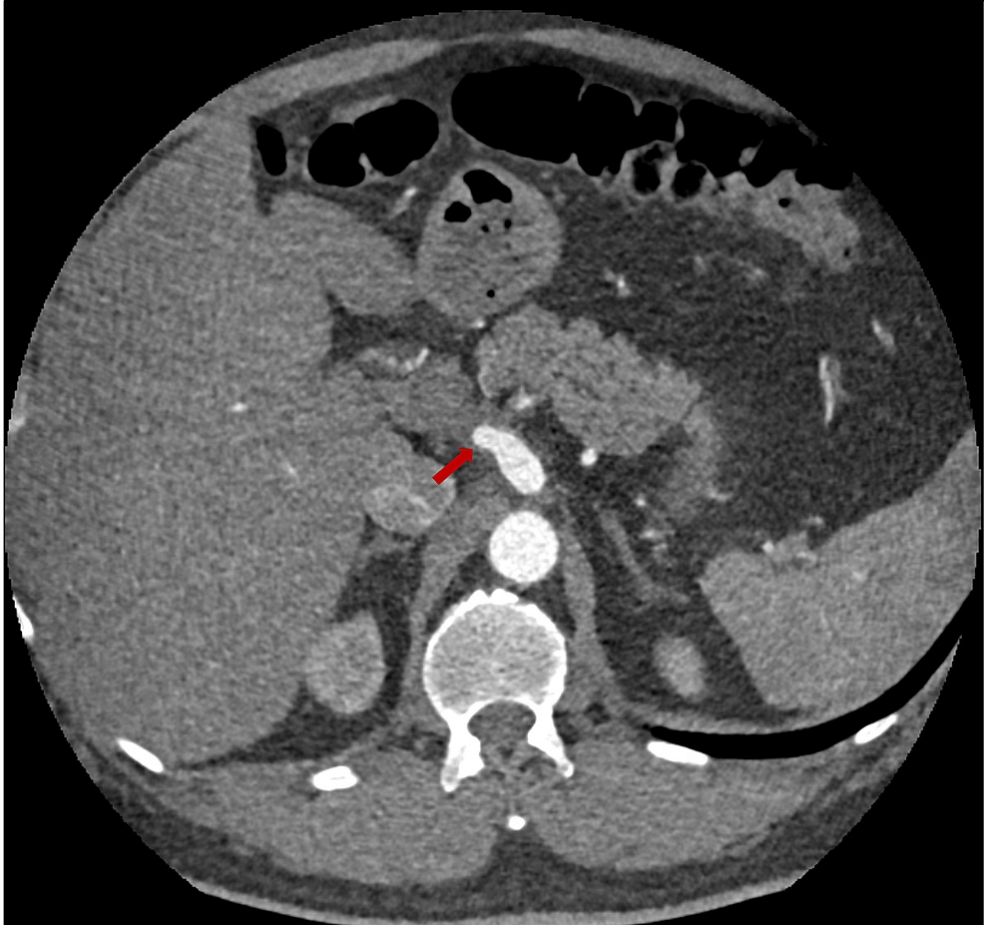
Axial contrast-enhanced CT scan of the abdomen showing abrupt cutoff at the common hepatic artery (red arrow).
